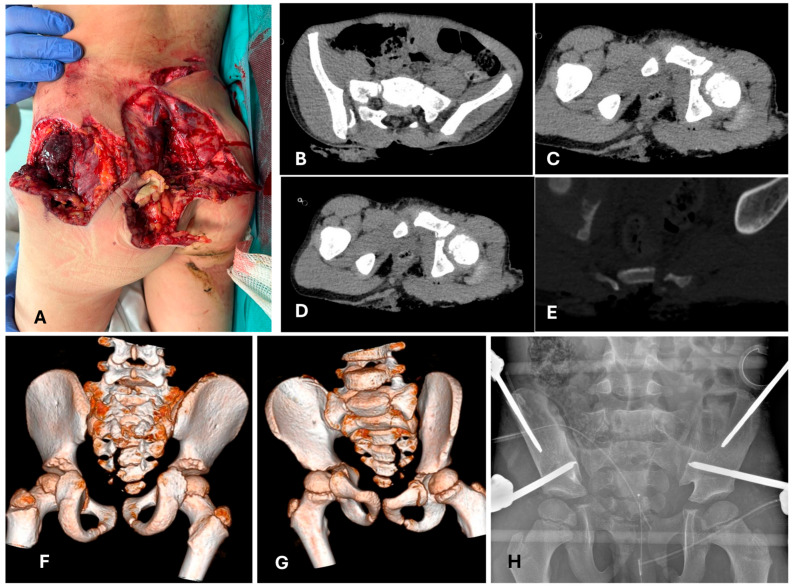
A 7-year-old patient, pediatric major trauma (PMT), accidentally run over by a snowcat. (A) Clinical presentation of the open sacral and pelvic fractures (back-view). (B–E) Computed tomography at admission; triplanar and (F,G) 3D reconstruction. Note the avulsion of the Risser from the right side, which corresponds to the closed book side of the pelvis, and the open sacral fracture, as described by Oransky et al. [25]. (H) Systemic (and local) damage control with temporary external fixation of the pelvis. A supra-acetabular and iliac configuration has been chosen according to both the instability of the patient and the local soft tissue situation.
